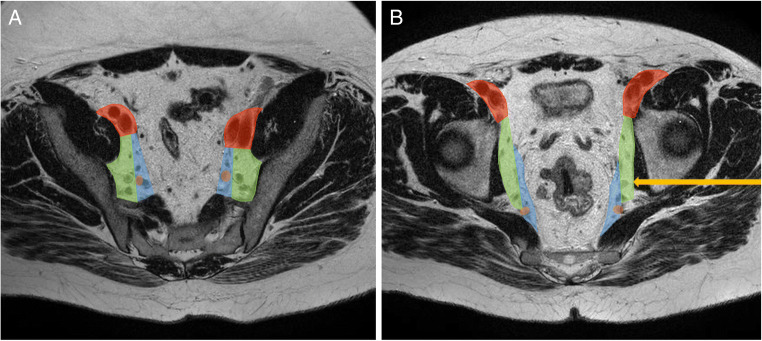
A, B Axial T2-MRI with colour atlas overlay depicting the lateral compartments. A Red—external iliac compartment, green—obturator compartment, blue—internal iliac compartment, orange spot—internal iliac artery, of which the lateral side of the main trunk forms the border between the obturator and internal iliac compartments. B Lateral lymph node, indicated with a yellow arrow
